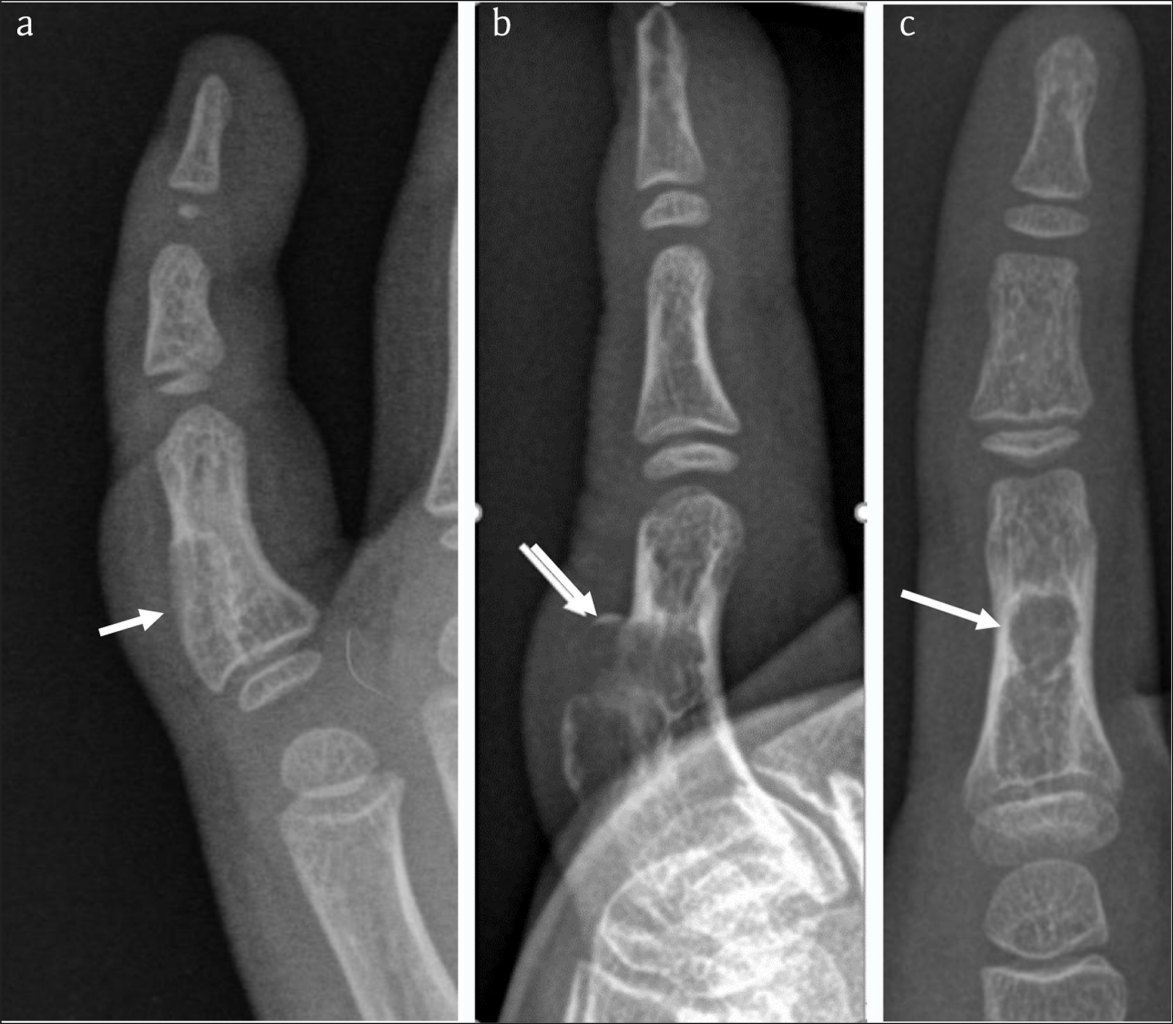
Case 1 – Conventional radiographs of the right fifth finger in a nine-year-old boy. Oblique view taken five years prior to the current admission (a). The lesion (arrow in a) was originally interpreted as a non-ossifying fibroma. Lateral (b) and anteroposterior (AP) view at current admission (c). The lesion has grown and consists of two components. The largest juxta-cortical and exophytic part causes pressure erosion of the dorsal cortex, which is thinned and focally destructed with overhanging edges (arrow in b). There is an associated soft tissue swelling. The smaller intramedullary part consists of a well-delineated radiolucent lesion with sclerotic borders. The latter is best seen on the AP view (arrow in c).
